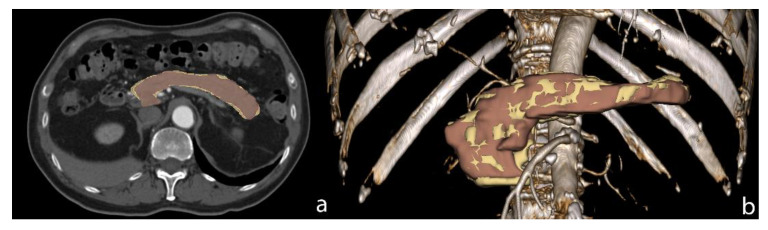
Pancreas AI segmentation (yellow) versus manual segmentation (brown) on axial CT image (a) and Volume Rendering image (b). The DSC of this case was 0.86.
